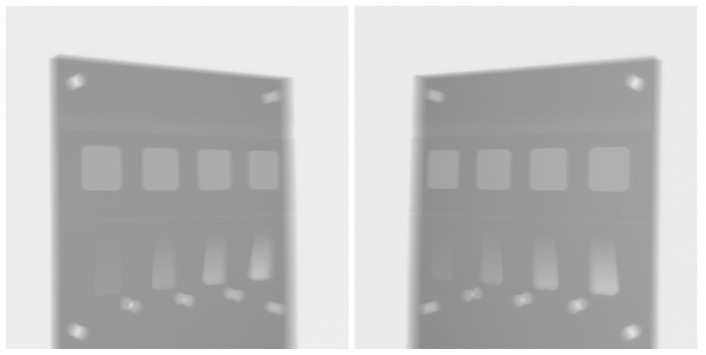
Intensity projection images of the physical test sample, collected by a Nikon XTH225 X-ray tomography system with a 60° relative rotation.
